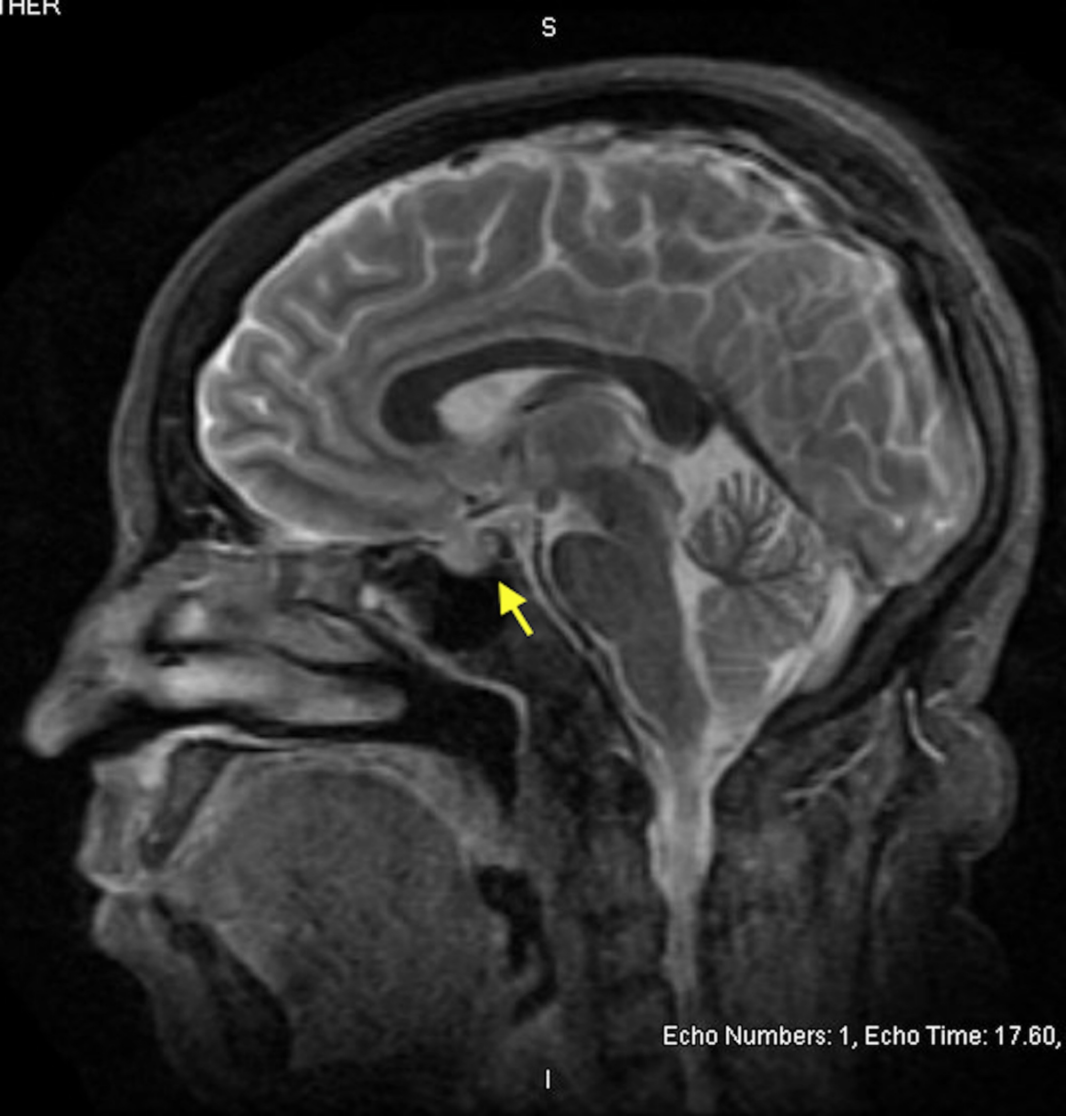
MRI brain in sagittal section showing abnormal enlargement of the pituitary gland (yellow arrow) with extension into the supra-stellar cistern abutting the undersurface of optic chiasm.
